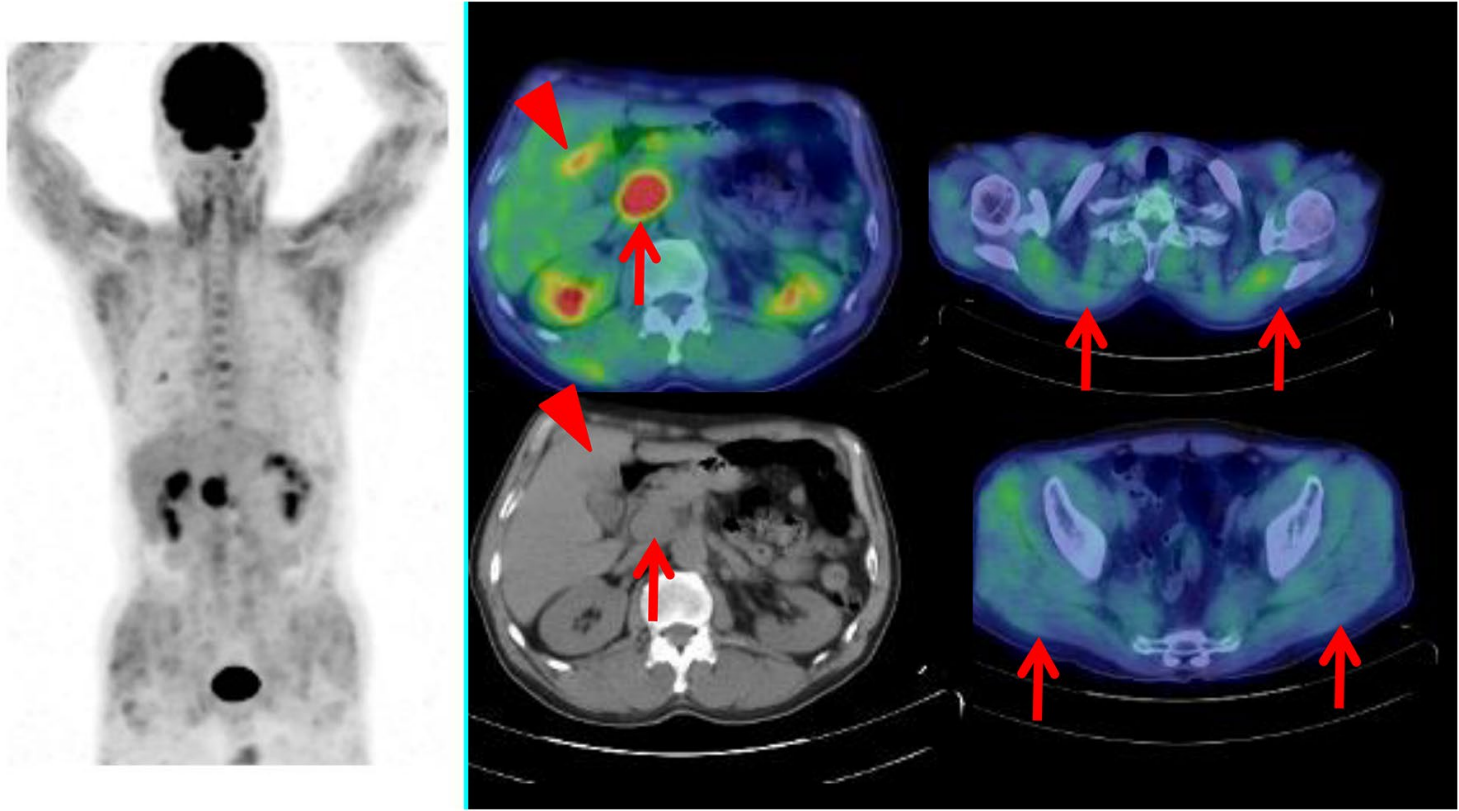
A dusky red rash developed systemically in the skin with itch in a 63-year-old male. Whole-body FDG PET maximum projection image showed heterogeneously increased FDG uptake in the systemic muscles. Cross-sectional FDG PET/CT images showed FDG-avid (SUVmax 3.1) gall-bladder cancer (arrow head) and metastatic lymph node near the root of the superior mesenteric artery (arrow), with heterogeneous but almost symmetrically increased FDG uptake in the muscle tissues (arrows)
